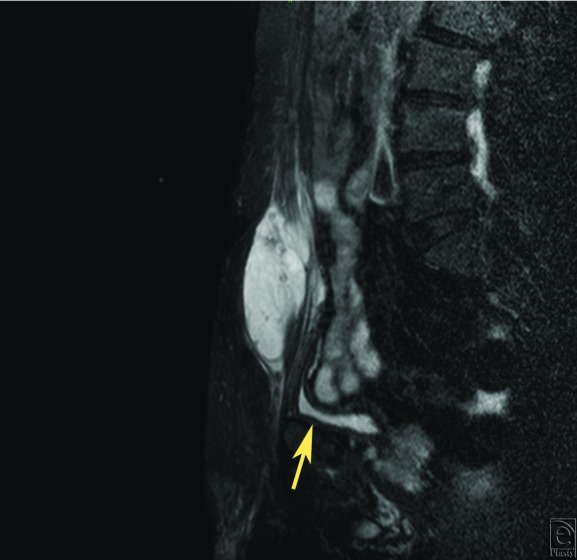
(a). Axial section of gadolinium-enhanced T_1_-weighted magnetic resonance imaging. The gross tumor was located in the right rectus abdominis muscle and spread horizontally. (b). Sagittal section of T_2_-weighted magnetic resonance imaging. The abdominal tumor did not spread into the abdominal cavity. The yellow arrow indicates the urinary bladder.
